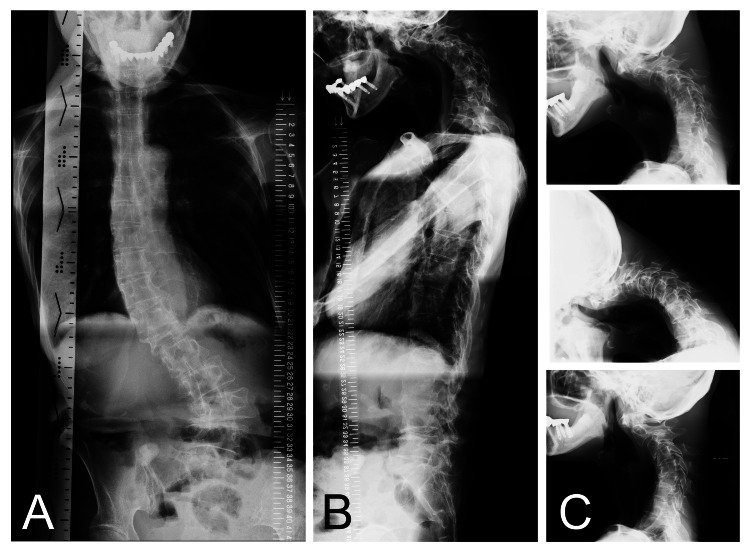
Plain radiographs of the spine of the same patient The view of the entire spine shows scoliosis in the antero-posterior view (A) and kyphosis in the cervical spine in the lateral view (B). The neutral position (C-top), flexion (C-middle), and extension (C-bottom) of the cervical spine are shown.
